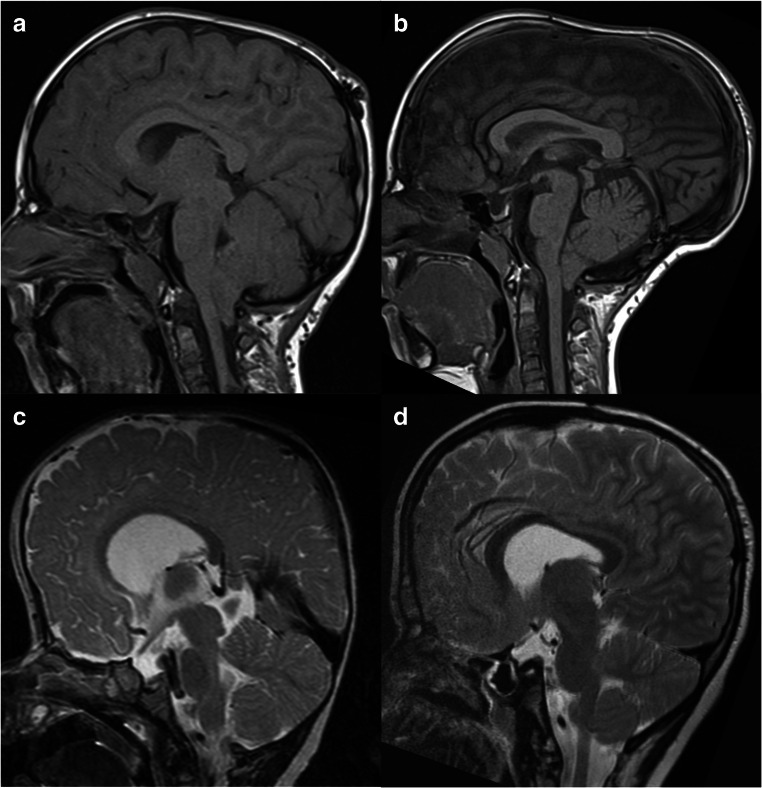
Pre-op and post-op mid-sagittal MRI images from a case of supratentorial (a, b) and infratentorial (c, d) PCD, from this study. Panels a and c show pre-op imaging for a supratentorial and infratentorial case respectively. Panels b and d show post-op imaging for the same supratentorial and infratentorial cases respectively. Pre-op images are from within 1 year of the operation date; post-op images are from at least 500 days post-op, when bone healing was certainly complete. Note in panel d the flattening of the tentorium, and compression/smoothing of the cerebellum, relative to the pre-op image in panel c—a change not seen between panels a and b, where the tentorium rises post-operatively (note the angles between the inner table and the superior aspect of the tentorium)
